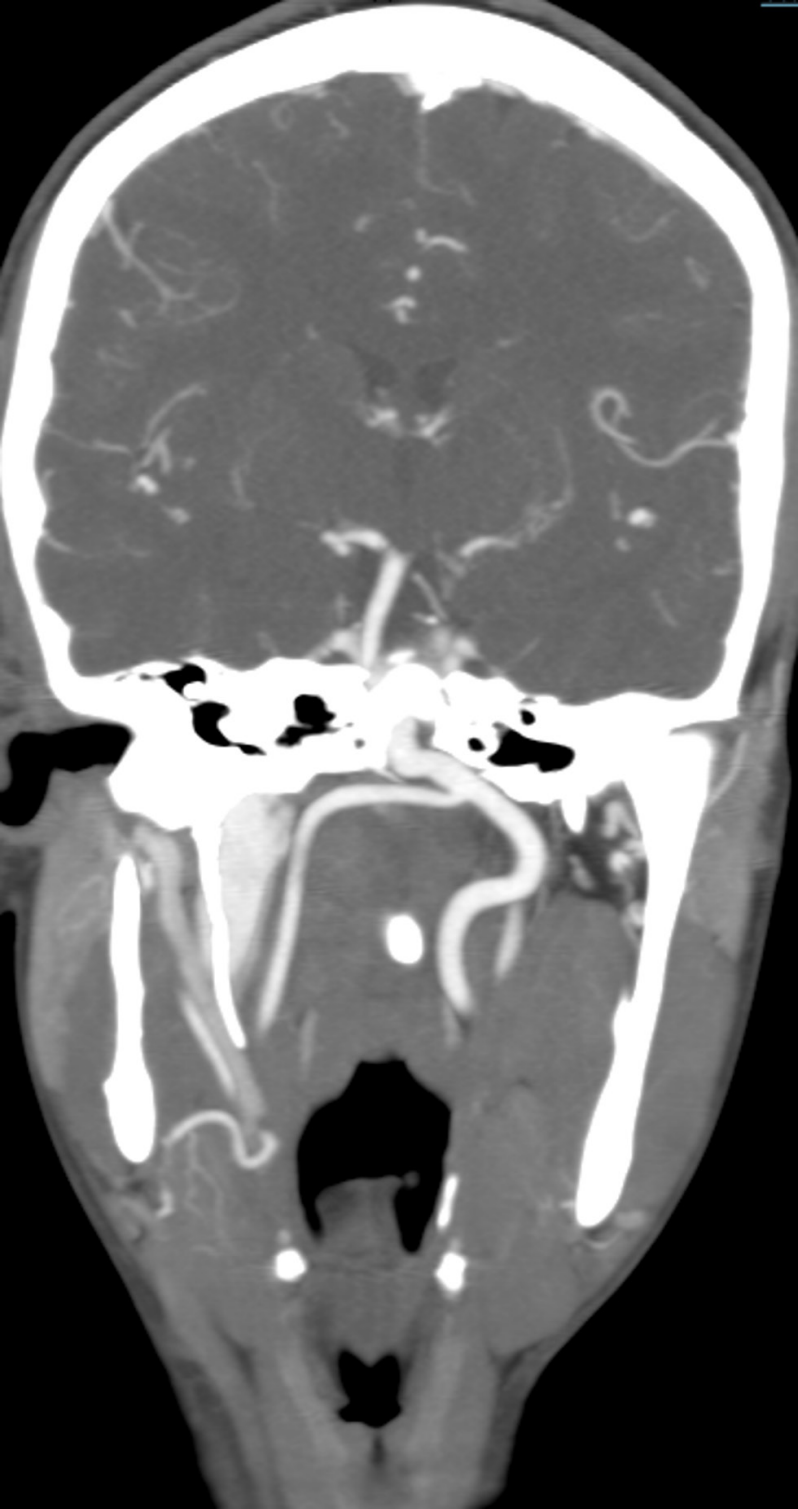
Coronal MIP CTA through the base of the skull shows the extracranial (ICA) to be tortuous, with right crossing to the left and fusing with the contralateral carotid in the retropharyngeal space. CTA, CT angiography; ICA, internal carotidartery; MIP, maximum intensity projection.
